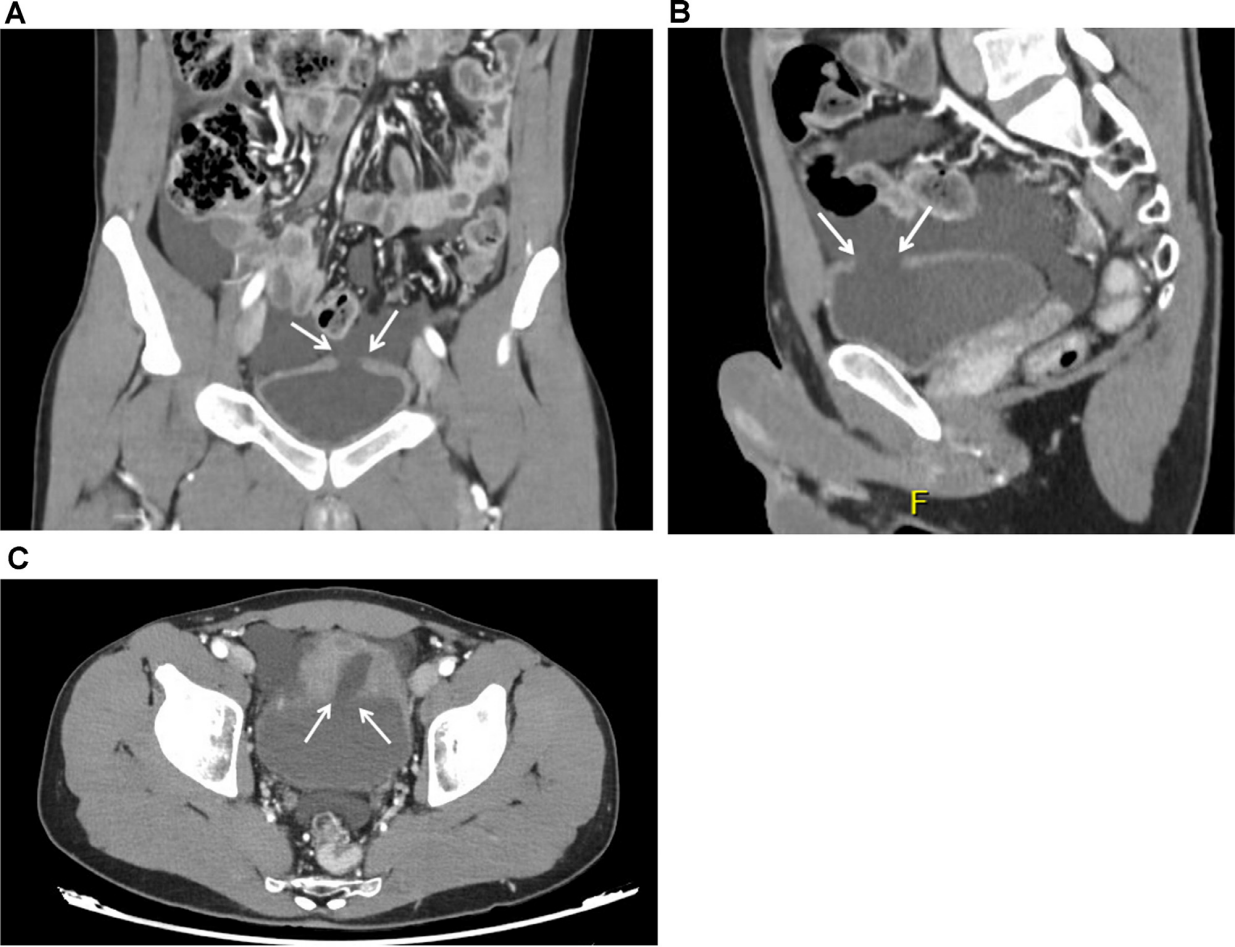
Contrast-enhanced CT of the small pelvis showing rupture of the dome wall of the urinary bladder (indicated by white arrows). Coronal (A), Sagittal (B), Axial (C).
